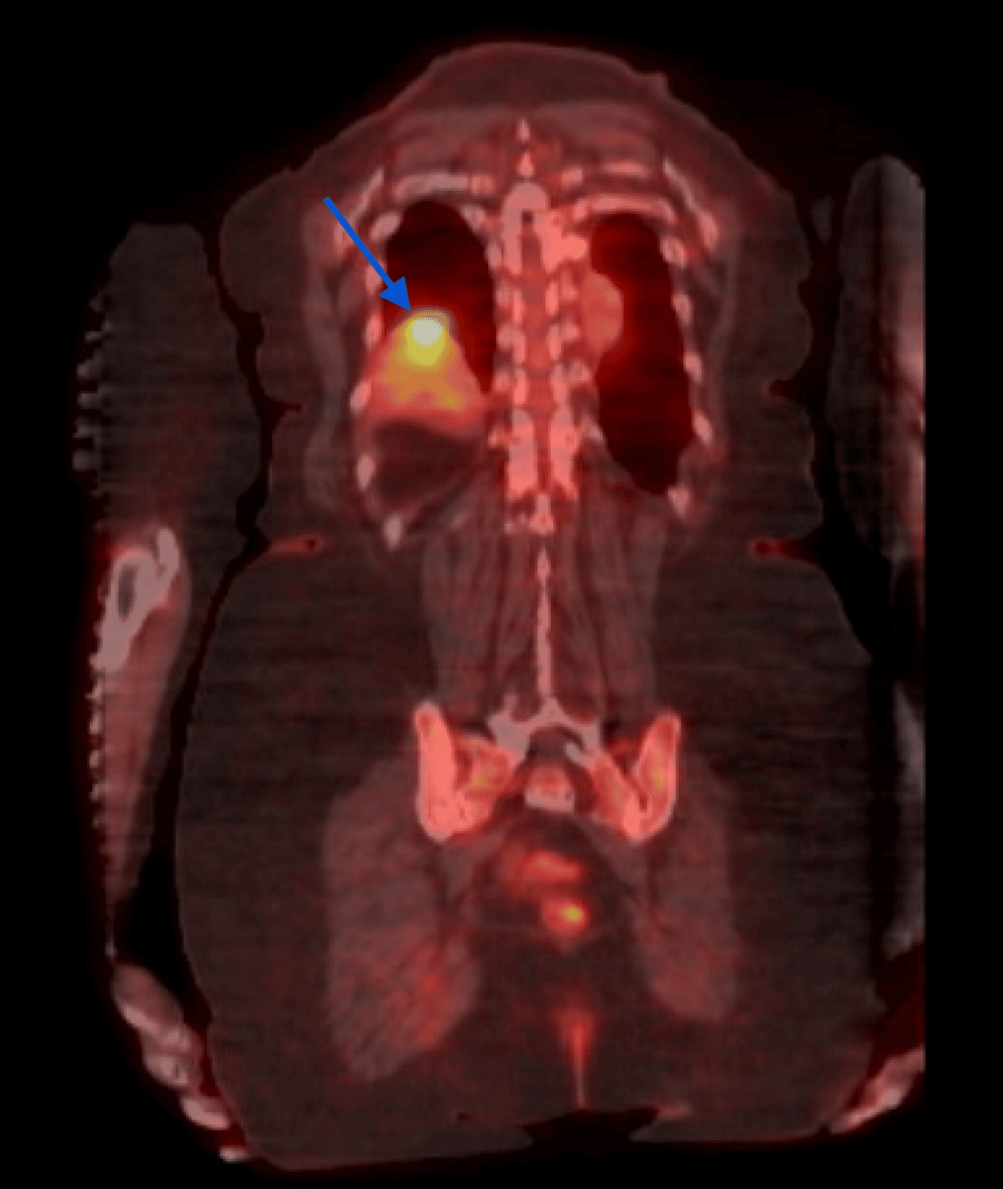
Whole-body PET/CT revealing a metastatic lung nodule. The blue arrow indicates metastatic lung nodule.
